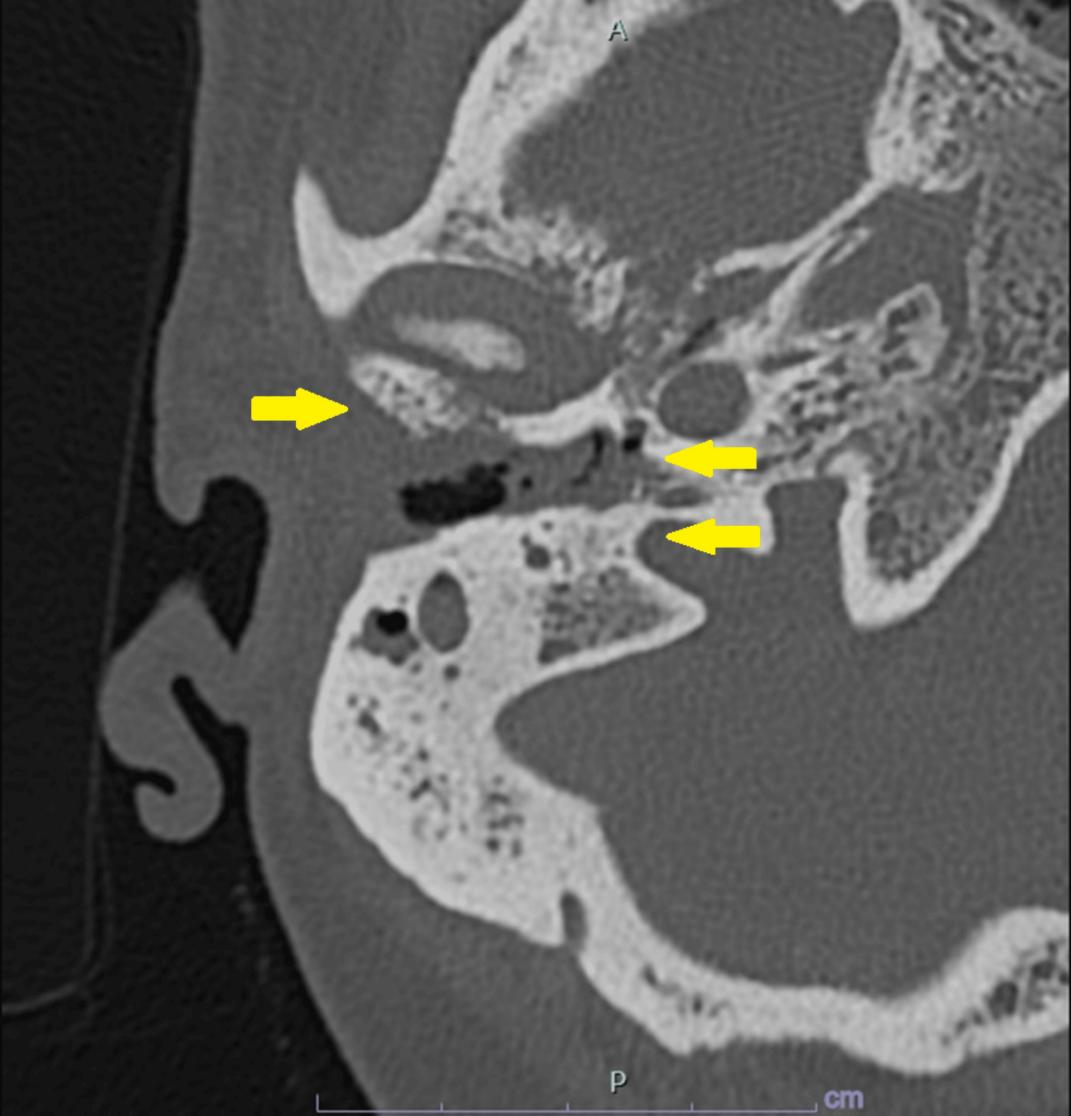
High-resolution CT scan of the temporal bone showcasing the osteolytic lesion, soft tissue invasion in the middle ear and mastoid.
